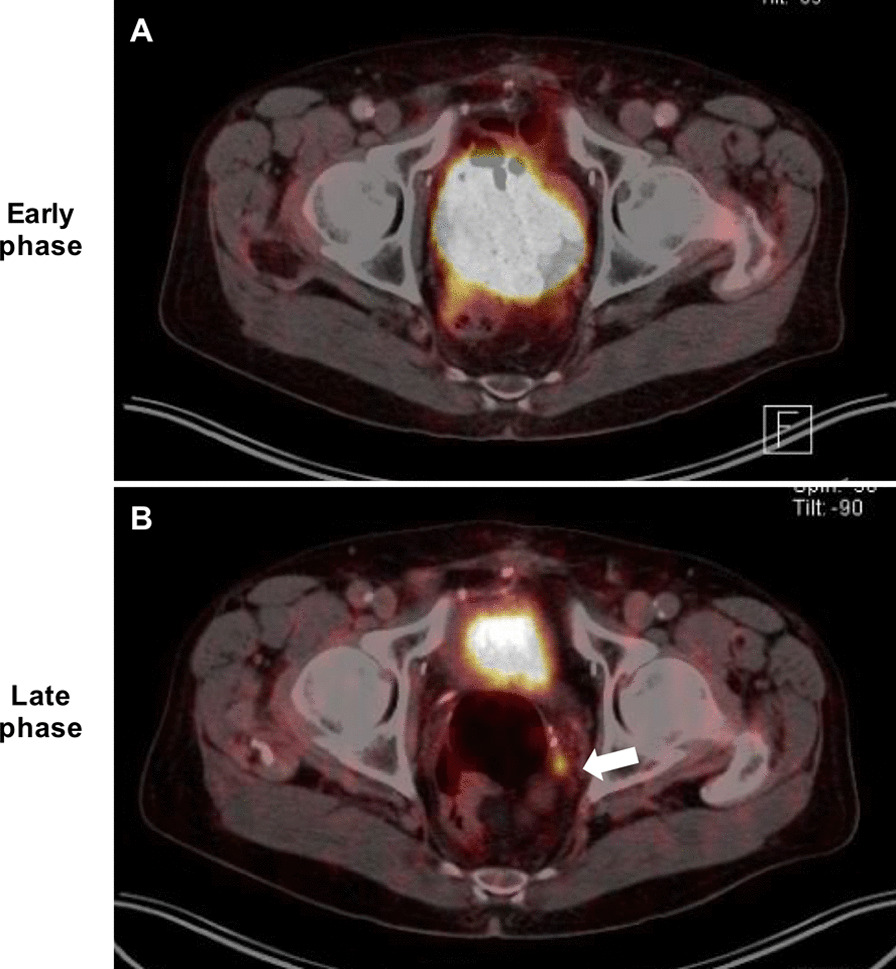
Example of a 81-year old patient with biochemical recurrence (PSA 1.5 ng/ml at the time of imaging. Para-rectal local recurrence (white arrow) was detected in late phase ^68^ Ga-PSMA PET/CT (B) because of enhanced tracer uptake (SUVmax 18.3), but was PET-negative in early phase^68^Ga-PSMA PET/CT (A). The suspected diagnosis of local recurrence was confirmed by follow-up multiparametric pelvic MRI
